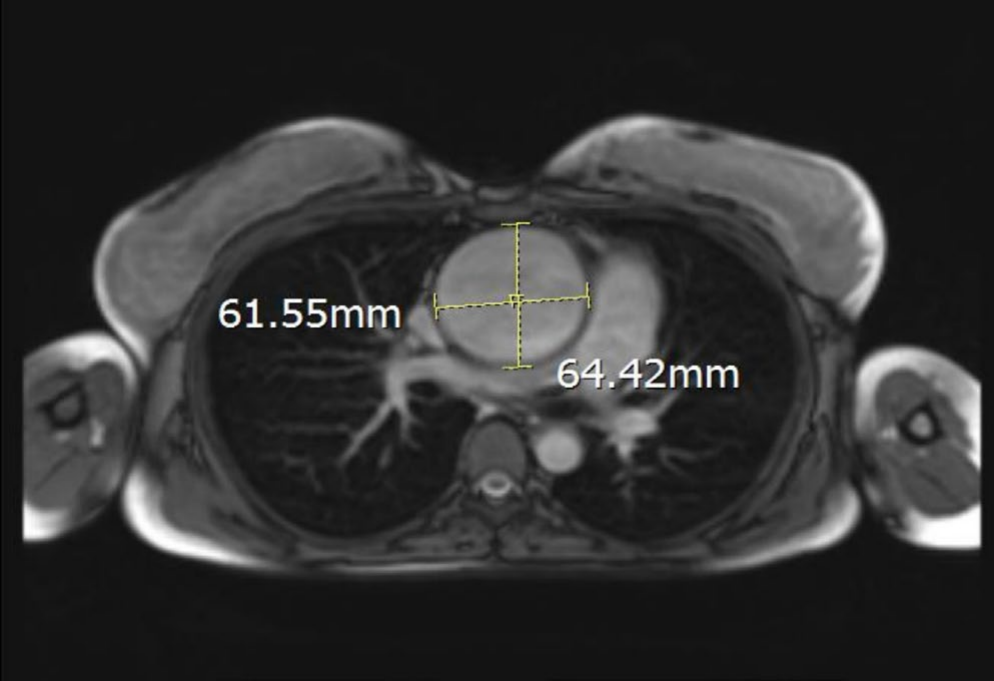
Axial view of thoracic magnetic resonance image without contrast during pregnancy.
